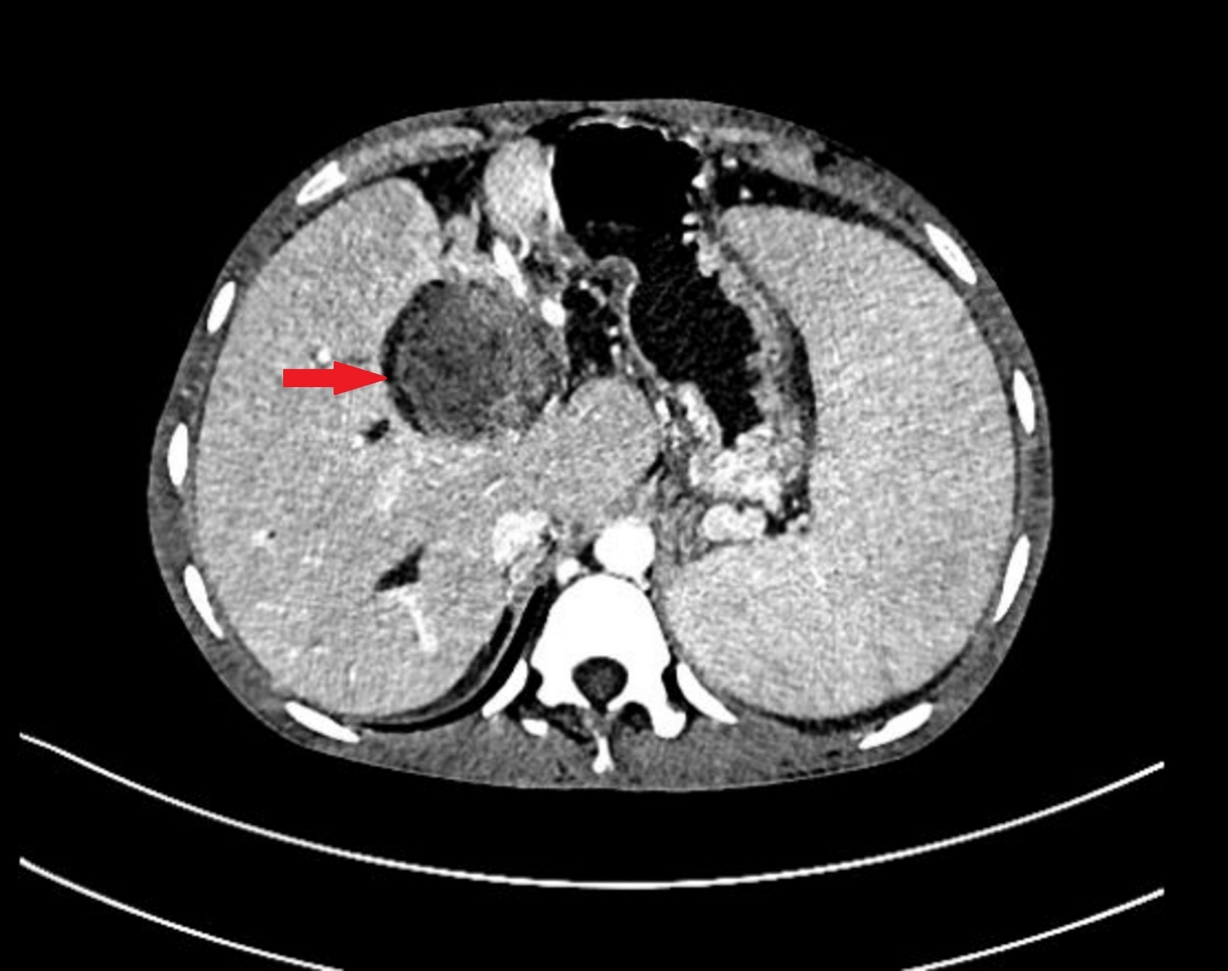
Hydatid cyst in the common bile duct on CT CECT abdomen axial image shows a well-defined rounded heterogeneously hyperdense lesion (red arrow) with peripheral calcification at porta. CECT: contrast-enhanced computed tomography, CT: computed tomography
